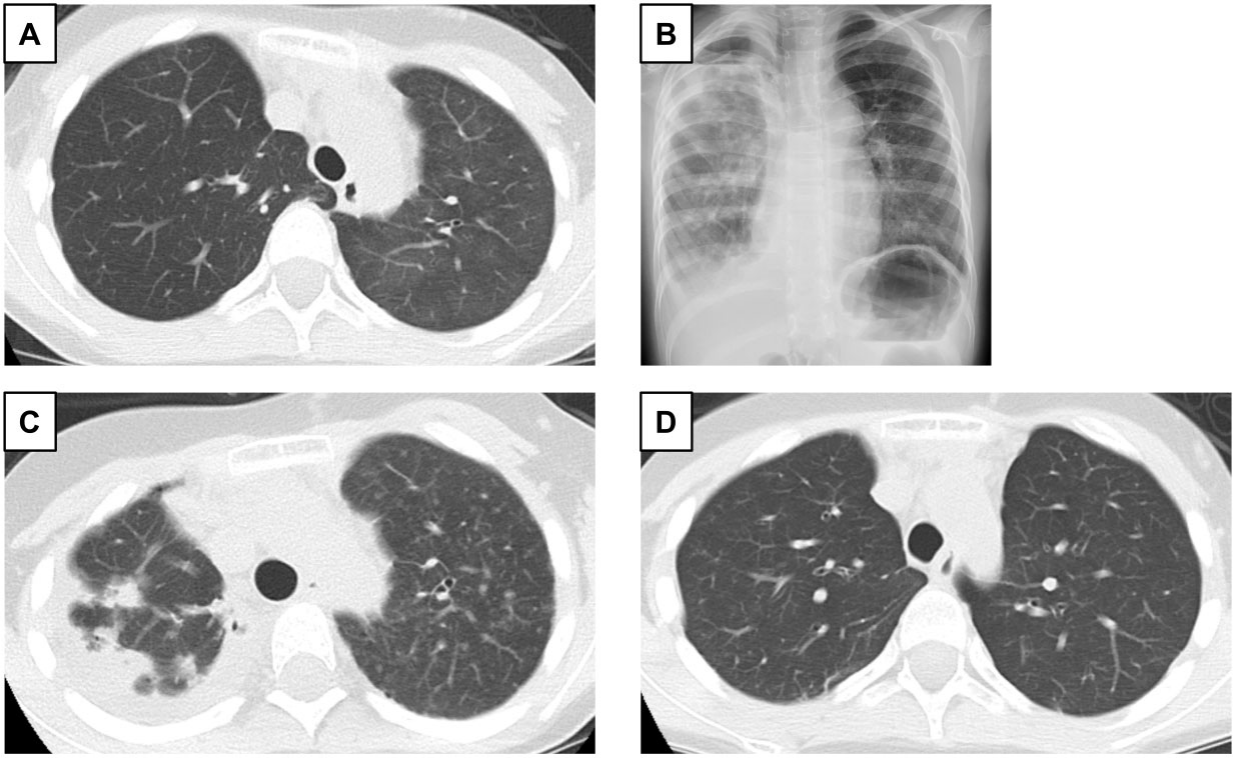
Chest computed tomography (CT) 2 months after right living donor lobar lung transplantation (LDLLTx) for idiopathic pulmonary arterial hypertension showing a right clear transplanted lung and a left native lung filled with slight ground-grass opacities (A). Chest X-ray 20 months after right LDLLTx showing broad infiltration in the right transplanted lung with pneumothorax (B). Chest CT 20 months after right LDLLTx showing chronic lung allograft dysfunction with subpleural fibrosis of the right lung and exaggerated ground-grass opacities all over the left lung (C). Chest CT 6 months after bilateral lung retransplantation from a deceased donor with downsizing bilateral lower lobectomies showing a clear lung field on both sides (D).
